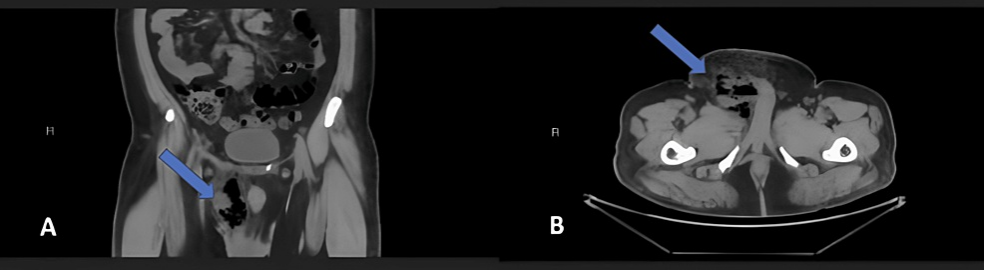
(A) Coronal view of the CT showing mixed air and fluid density seen along the right spermatic cord course and the right epididymis with the air locules seen exceeding the right inguinal canal and the pubic area with subcutaneous air and fat stranding at the right pubic region and (B) axial view showing the same finding.
